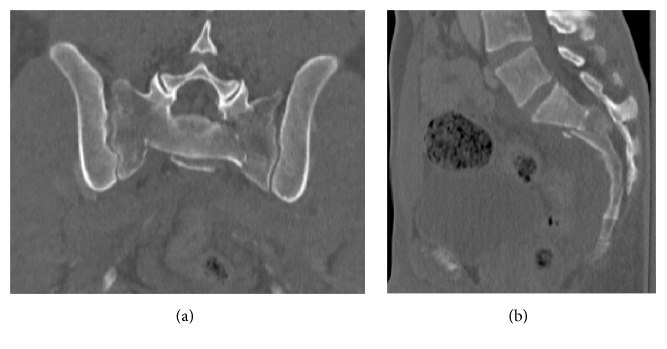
Coronal (a) and midsagittal (b) preoperative CT scan. Note the bilateral sacral fractures with horizontal S2 fracture with a 37-degree kyphotic deformity.
